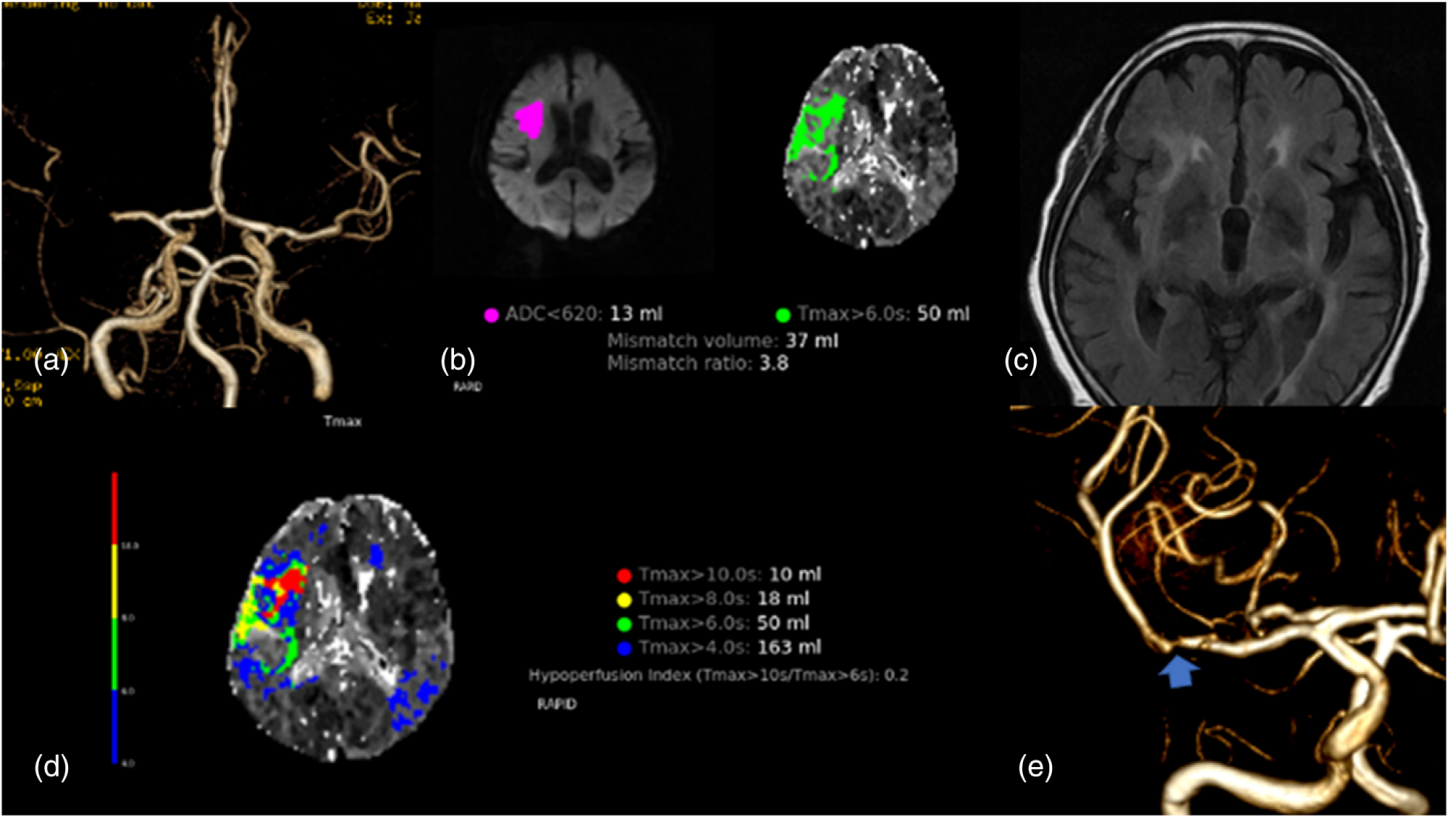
(A) MR angiography. (B) DWI‐PWI map. (E) FLAIR imaging. (D) *T*max map. (E) MR angiography before onset.
